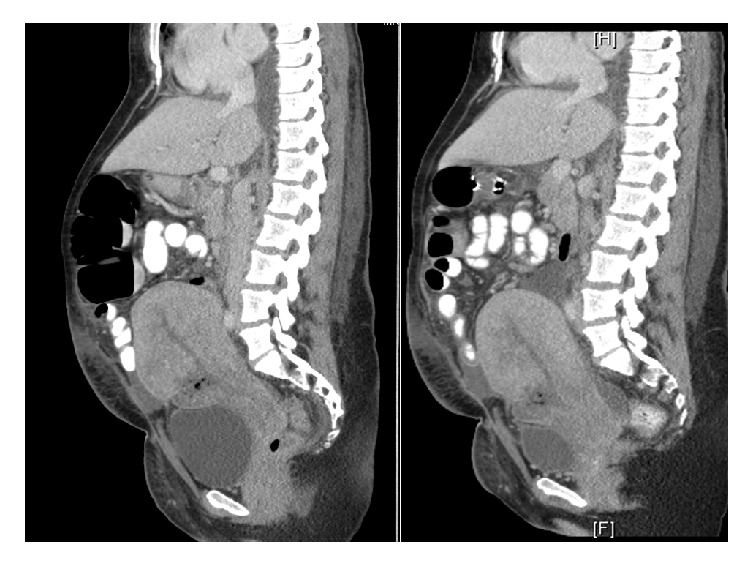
On the left, there is a CT A/P with IV contrast in sagittal view demonstrating that the uterus is enlarged and heterogeneously consistent and an approximately 2.6 x 2.5 cm defect is noted at the C-section incision site containing hypodense fluid and multiple bubbles of gas. Also, a focus of gas is also noted within the endometrial cavity. On the right, there is a CT A/P with IV contrast in sagittal view done two days after the one on the left demonstrating a persistent 2.6 cm defect at the C-section incision site containing fluid and multiple bubbles of gas. Now this defect is continuous with a 3.3 x 0.9 cm abscess along the anterior aspect of the uterus.
